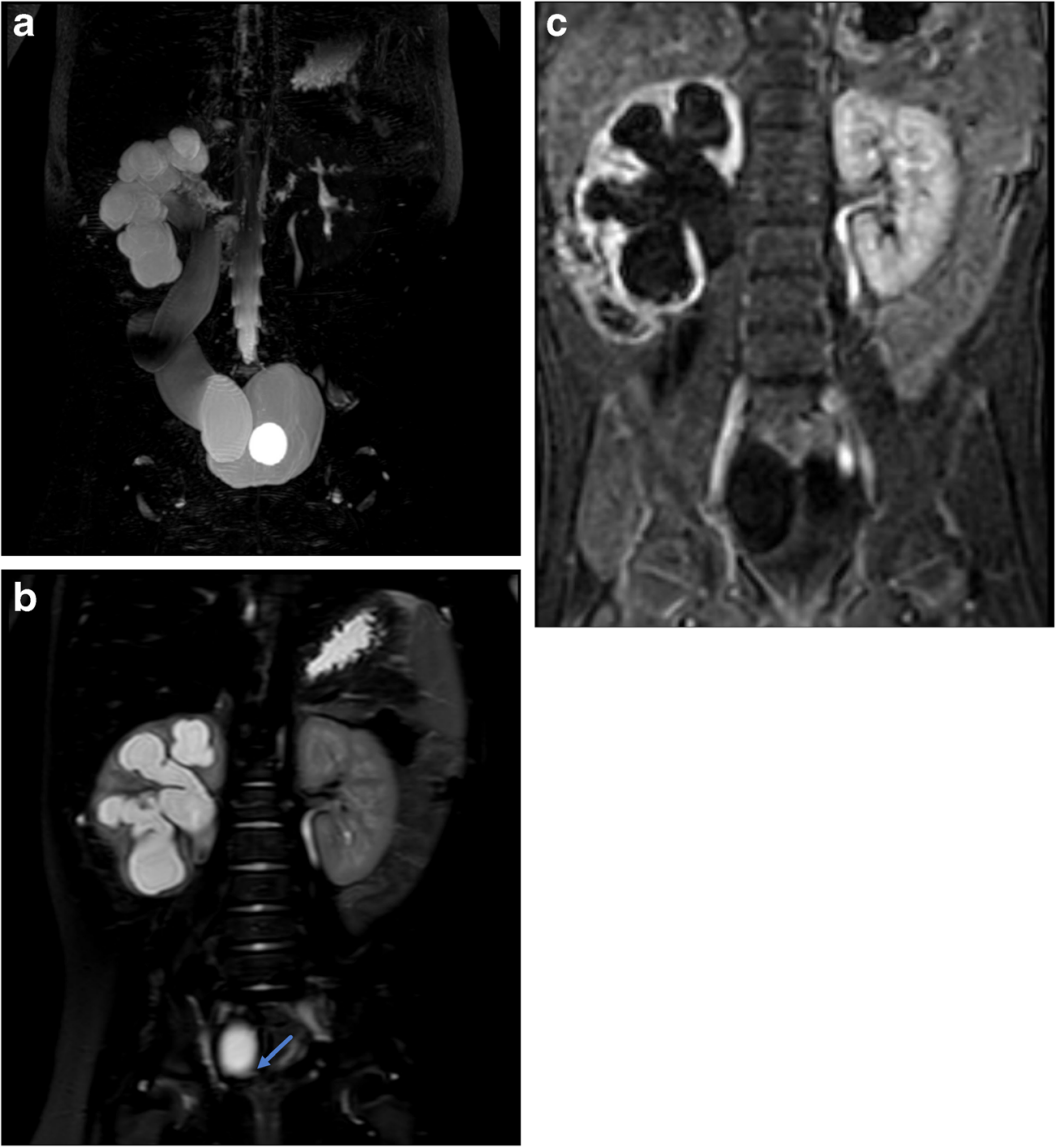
Six-month-old with history of febrile UTI. Initial ultrasound showed moderate to severe right hydronephrosis and megaureter. VCUG showed grade 3 left-sided reflux, no right reflux, and bilateral periureteral diverticula. Together with the MRU findings, this patient was diagnosed with refluxing, obstructive congenital primary megaureter. a Coronal maximum intensity projection (MIP) image from a 3D T2-weighted sequence shows marked right pelvocaliectasis and a dilated, tortuous right ureter. A bladder catheter is in place with a fluid-filled balloon in the bladder lumen. b Coronal T2-weighted single-shot fat suppressed image shows abrupt narrowing and “beaking” of the distal ureter at the ureterovesical junction consistent with obstruction. c Single image from the dynamic post-contrast sequence shows diffuse parenchymal thinning of the right kidney with delayed excretion into the renal collecting system relative to the left, indicative of clinically relevant obstruction
